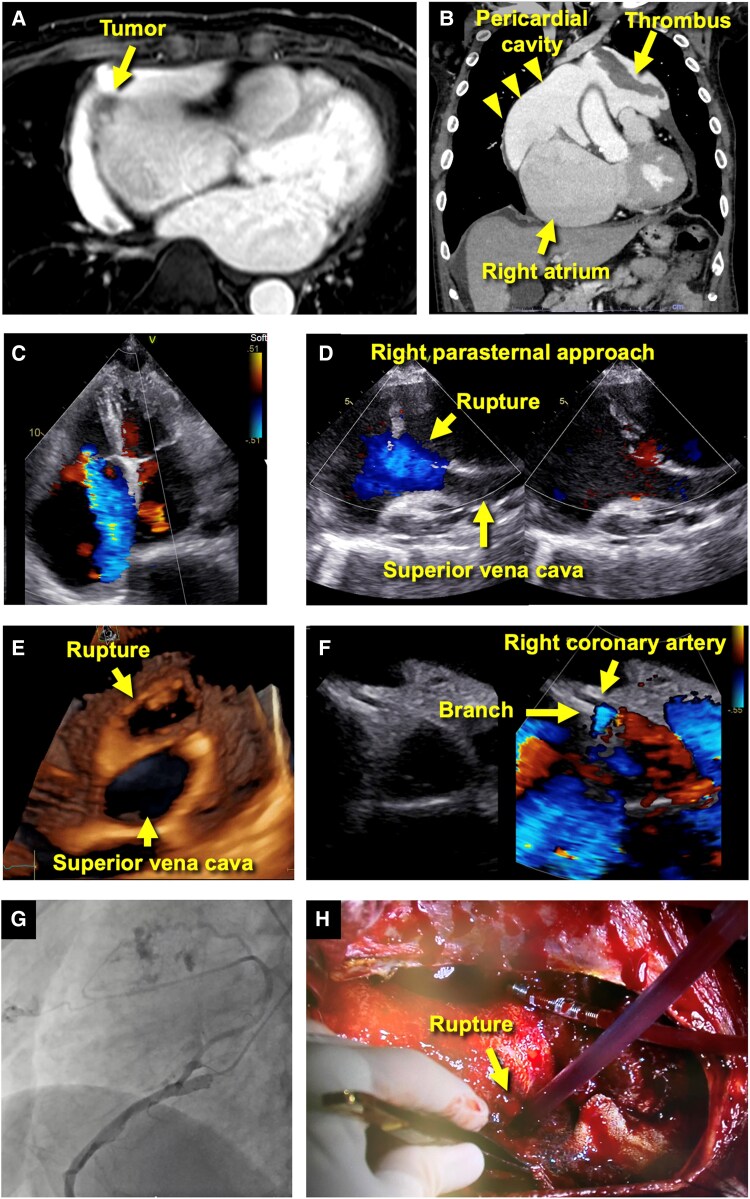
(*A* and *B*) Computed tomography (axial and coronal views) revealed a rupture in the right atrium along with pericardial effusion containing thrombus. (*C* and *D*) Transthoracic echocardiography in the apical four-chamber view failed to visualize the rupture but was identified in the right parasternal view. (*E*) Three-dimensional transthoracic echocardiography (right parasternal view) reconstructed an *en face* view of the rupture and a cross-section of the superior vena cava. (*F*) Parasternal short-axis view with colour Doppler detected high-flow signals in a branch of the right coronary artery, suggesting a fistula to the pericardial space. (*G*) Coronary angiography confirmed the presence of the fistula. (*H*) Surgery revealed the right atrial rupture, and the atrial wall was reconstructed.
